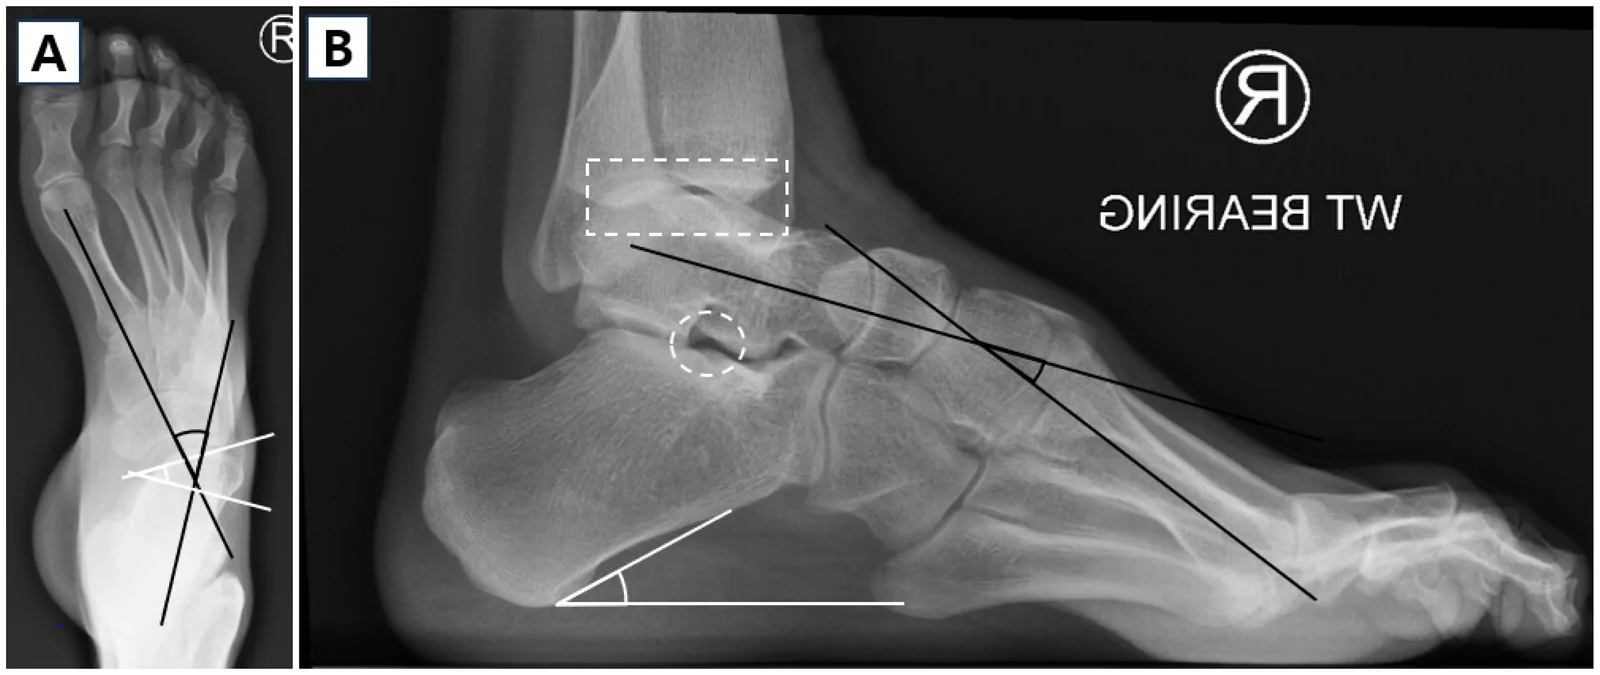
Radiographic measurements and characteristic signs used to evaluate CMT-related foot deformity. (A) Anteroposterior foot radiograph showing the axial Meary angle (black angle) and talonavicular coverage angle (white angle). (B) Lateral foot radiograph showing the sagittal Meary angle (black angle) and calcaneal pitch angle (white angle). The double talar dome sign (dashed rectangle) and sinus tarsi see-through sign (dashed circle) are also observed.
